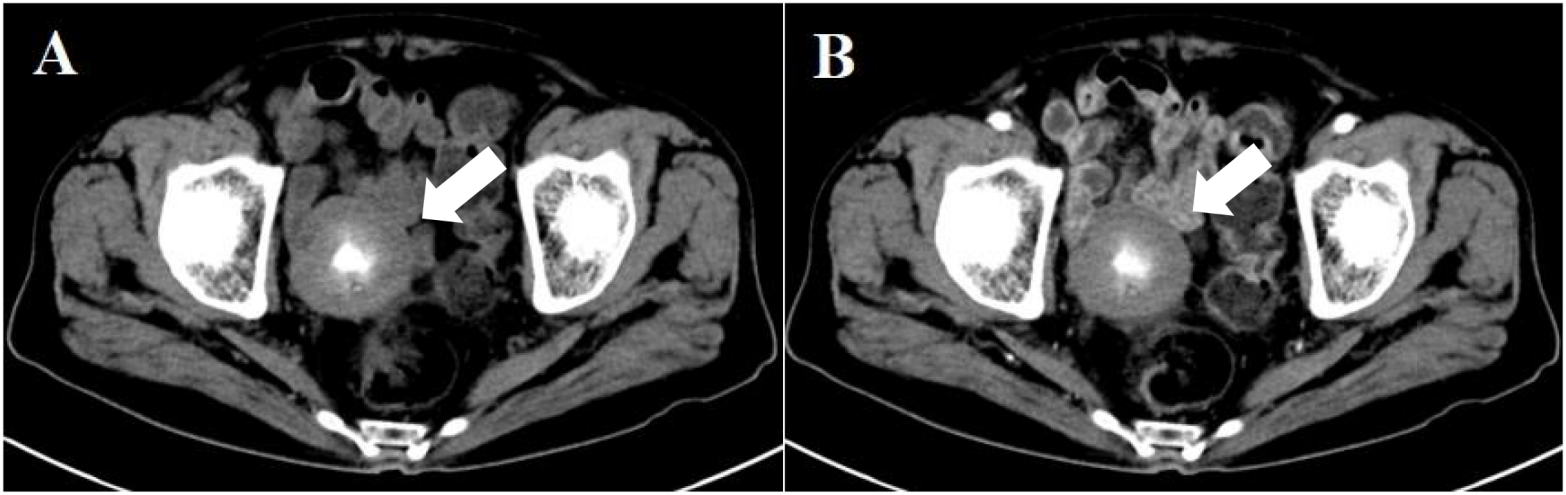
Computed tomography reveals a quasi-round mass (indicated by the white arrow), with soft-tissue density at the periphery and irregular central calcification (A). Contrast-enhanced CT shows no enhancement of the quasi-round mass (B).
